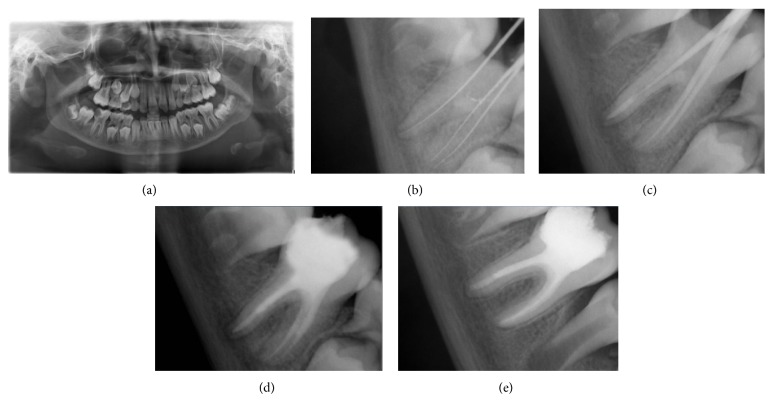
(a) Preoperative. (b) Working length determination. (c) MTA apical plug of 4-5 mm thickness. (d) Final obturation. (e) After 8-month reassessment, continuous lamina dura and consistent width of periodontal ligament space suggest healing of the periapical lesion.
